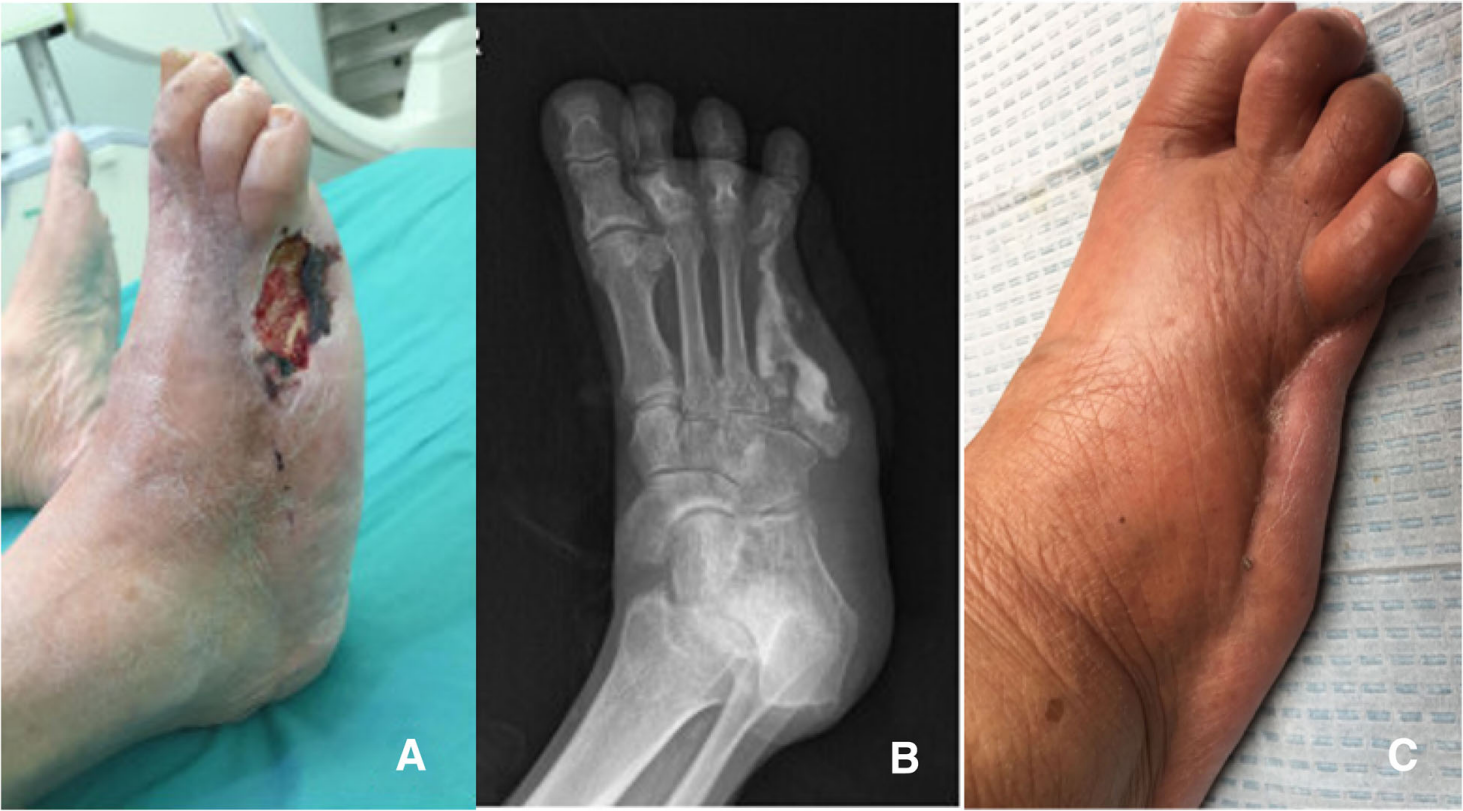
A patient with the fourth infected metatarsal (the fifth phalanx and metatarsal had been resected 5 years ago) were resected to the base of metatarsal before the vancomycin-impregnated calcium sulfate was injected into the dead space. a The presentation of wound before the operation. b The X-ray presentation 3 days after operation. c The ulcer had healed and no symptoms of osteomyelitis were presented 1 year after operation
